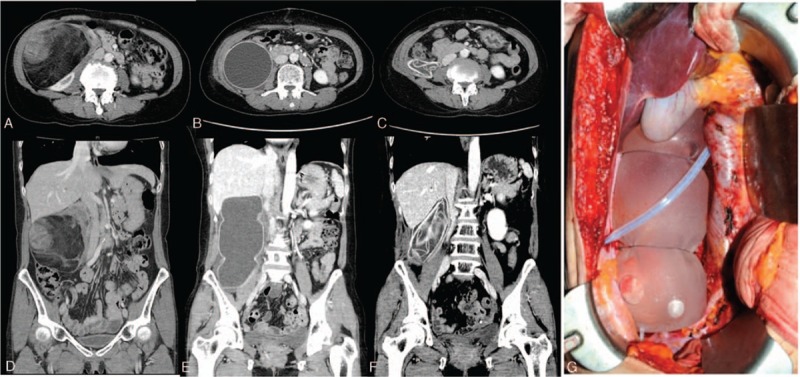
Representative computed tomography (CT) and intraoperative findings. A, Preoperative CT axial image. B, Postoperative (1 wk) CT axial image. C, Postoperative (2 yr 5 mo) CT axial image. D, Preoperative CT coronal image. E, Postoperative (1 wk) CT coronal image. F, Postoperative (2 yr 5 mo) CT coronal image. G, Intraoperative TE insertion finding. A 53-year-old woman underwent a mass excision with right nephrectomy of a dedifferentiated liposarcoma (FNCLCC grade 2/3). A tissue expander (760 mL) with injection valve was inserted and the patient was treated with postoperative radiotherapy (54 Gy). There was no evidence of tumor recurrence after 2 years and 5 months.
